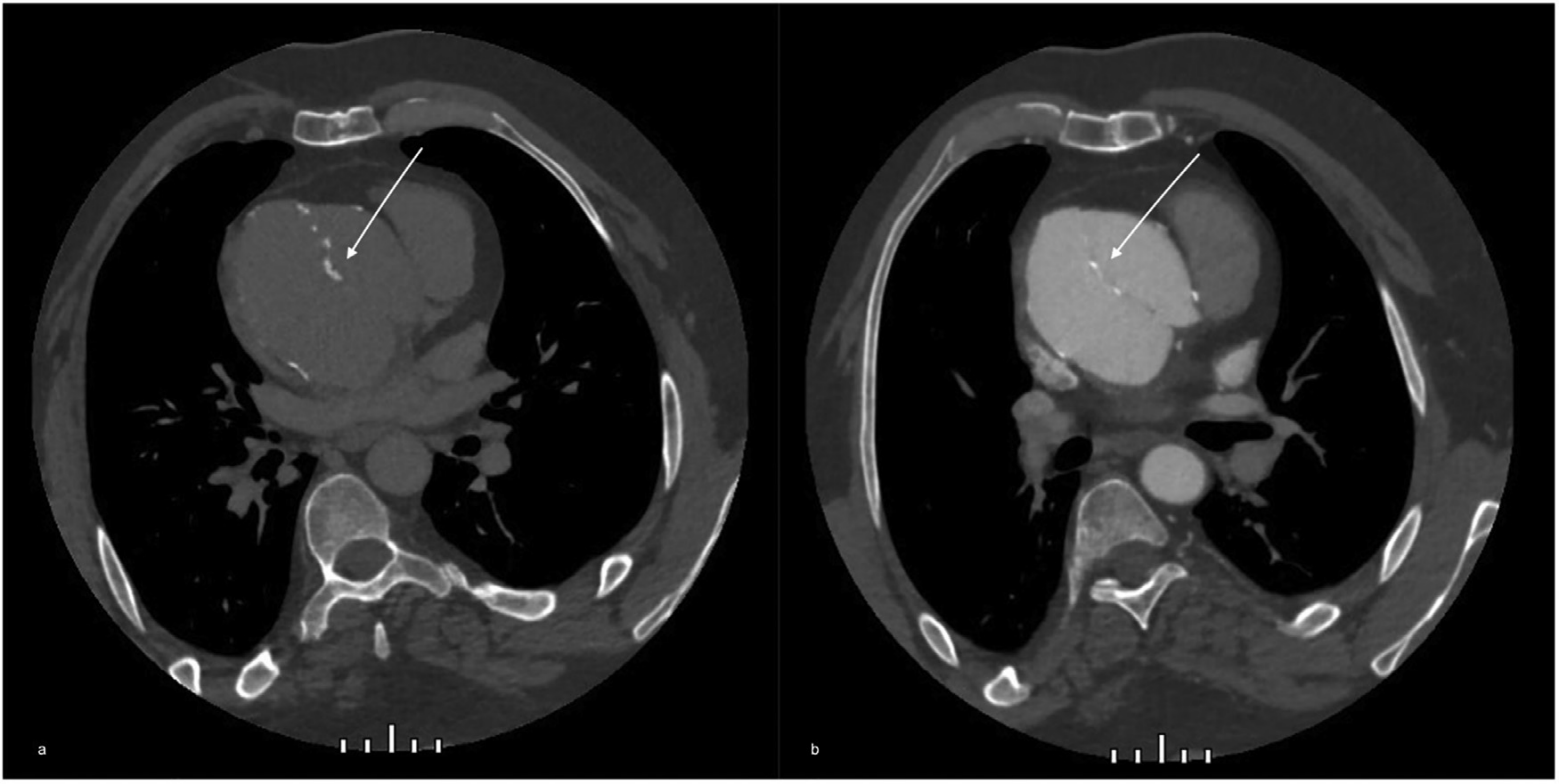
Unenhanced (A) and enhanced (B) axial image of the same preoperatory CT shown in Figure 2 with a focus on the “flap-like” aspect (white arrows). It can be seen a small septum with calcific spots that seemed to divide the aortic lumen into 2 cavities.
